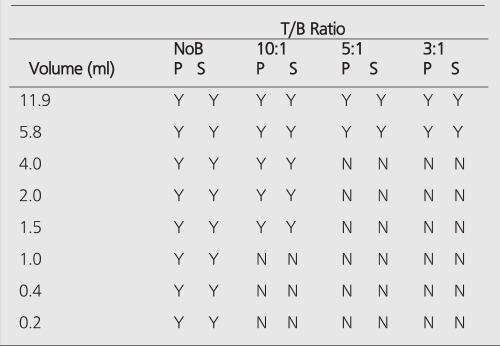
Limits of detection for I-131. Y denotes that sphere isvisible on planar (P) and SPECT (S) images, N means otherwise
